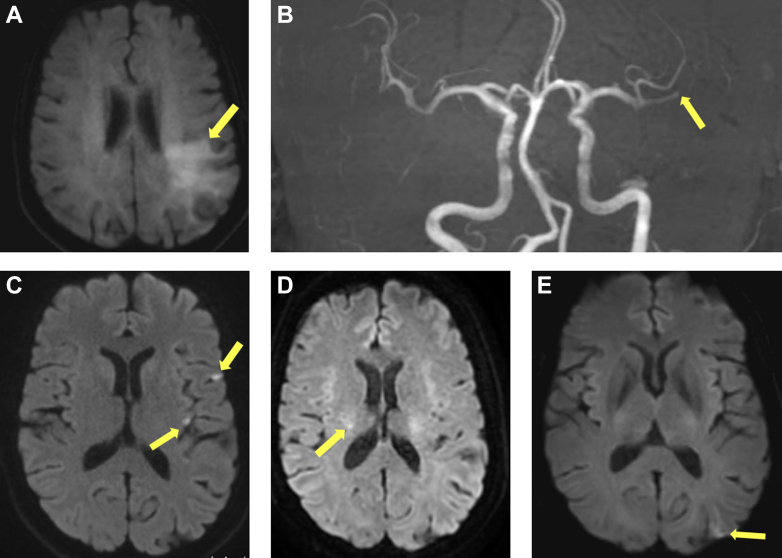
Brain Magnetic Resonance Imaging demonstrating Recurrent Multifocal Ischemic Events Brain magnetic resonance imaging (MRI) demonstrating multifocal ischemic events during the clinical course. A and B correspond to the initial stroke; C to E represent selected imaging from transient ischemic attack 5, 7, and 8. (A) Diffusion-weighted MRI showing acute ischemia in the left insular and parietal cortex. (B) MRI (time-of-flight angiography) demonstrating thrombus in the left M2 segment. (C) MRI revealing 2 of 3 silent cortical infarcts in the left hemisphere. (D) MRI showing punctate diffusion restriction in the right corona radiata. (E) MRI showing cortical diffusion restriction in the left hemisphere. M2 = 2nd segment of the middle cerebral artery.
